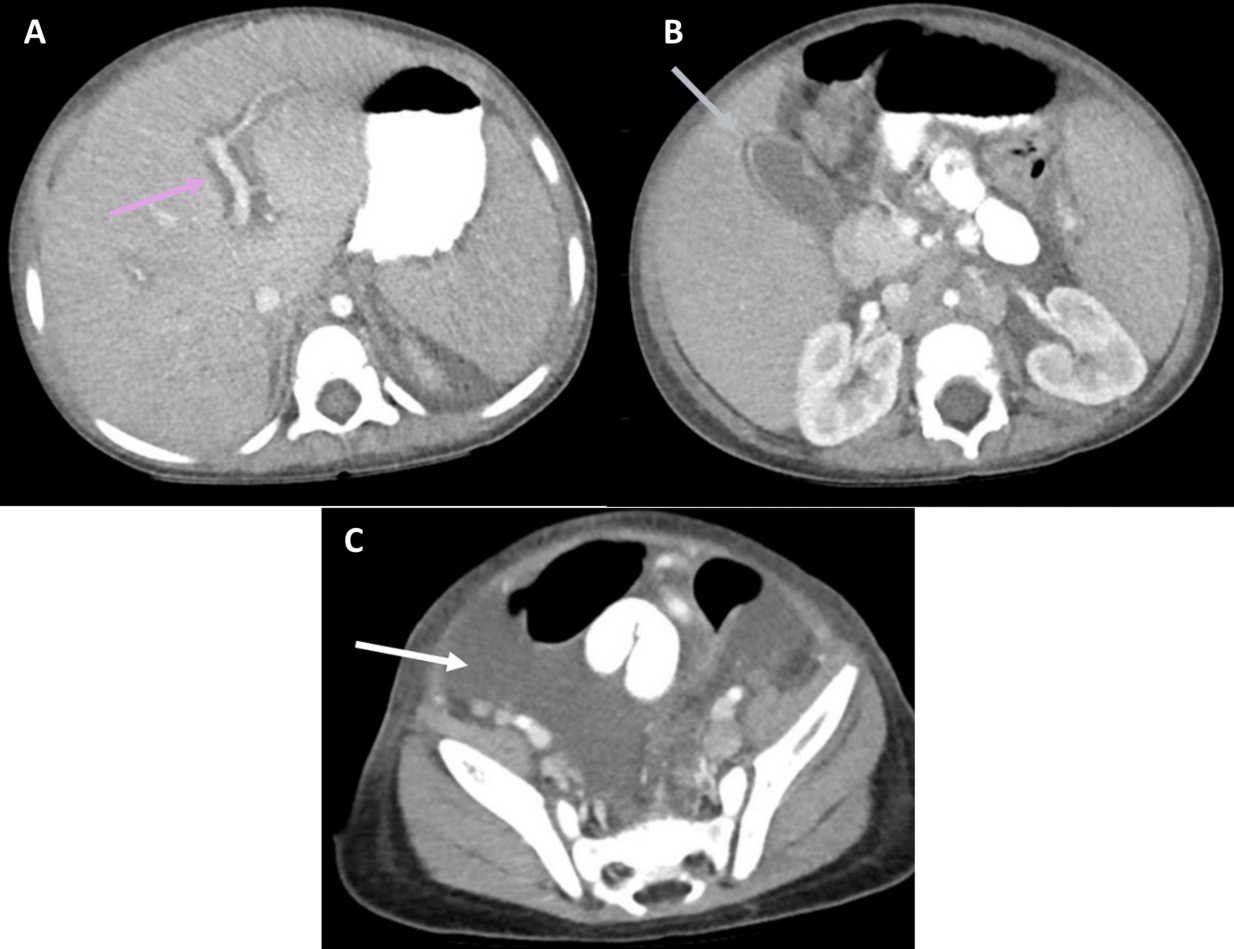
A two-year-old with HLH and multiorgan failure CT with IV contrast demonstrates peri-portal edema (arrow) (A), gallbladder wall thickening (arrow) (B), and ascites (arrow) (C). HLH: hemophagocytic lymphohistiocytosis
